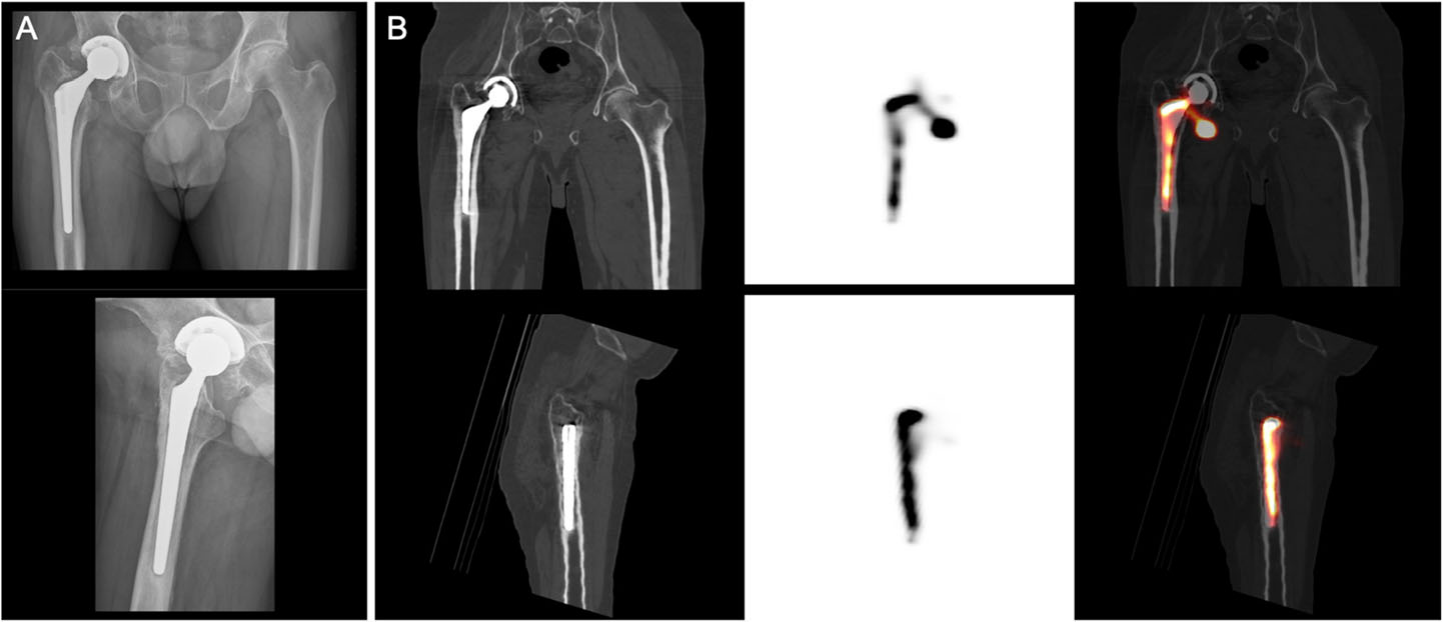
Femoral component loosening of a right hip total arthroplasty. The radiograph images demonstrate lucency along the metal-bone interface (first column). Coronal (top) and sagittal CT (second column), SPECT (third column), and fused SPECT/CT (fourth column) images showing abnormal tracer activity along the bone-prosthetic interface of the femoral component
